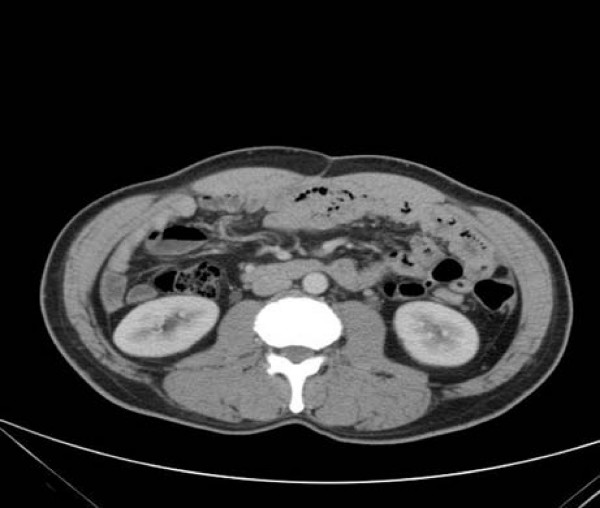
CT scan of abdomen – postoperative 17 months, compared with Fig 1. No local recurrence or metastatic lesion is identified.
